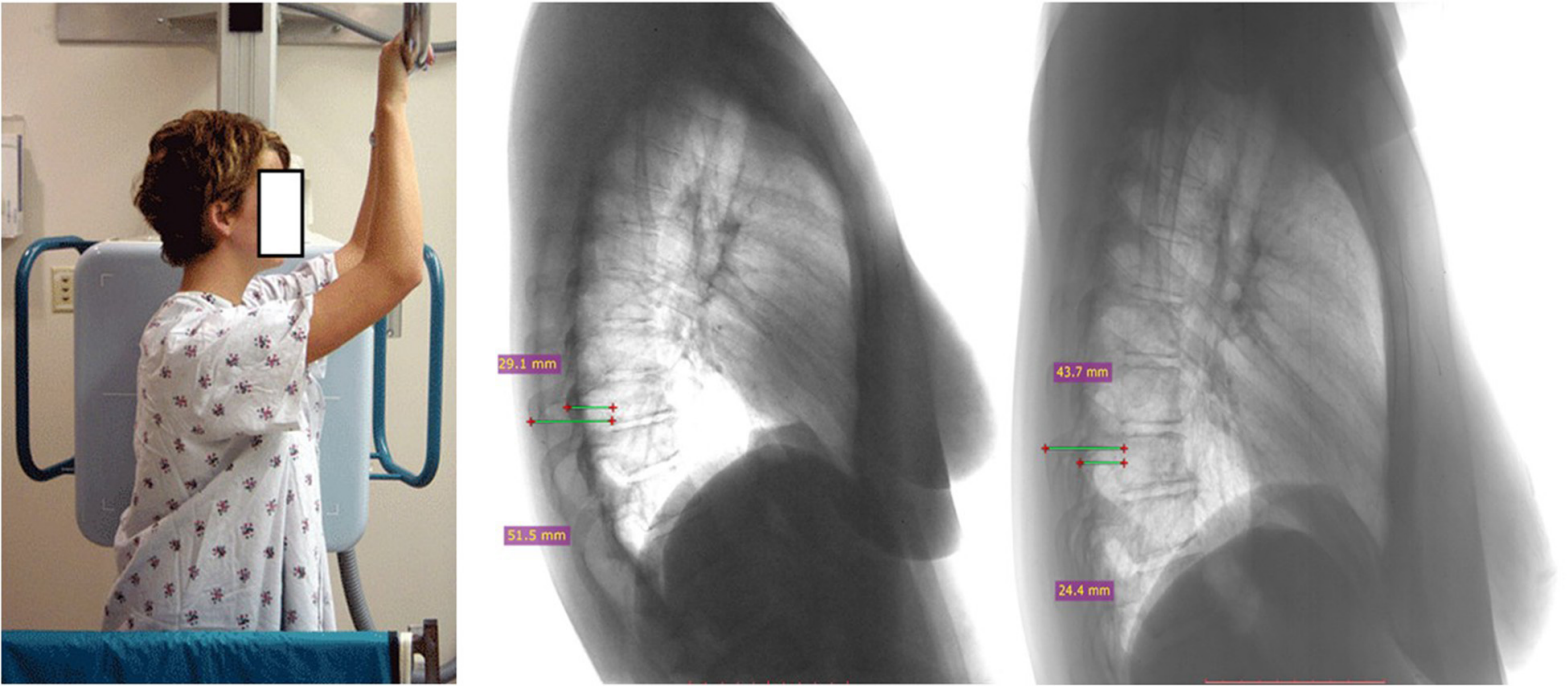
The radiographs were obtained in a standard position. Measurements in two radiographs.
